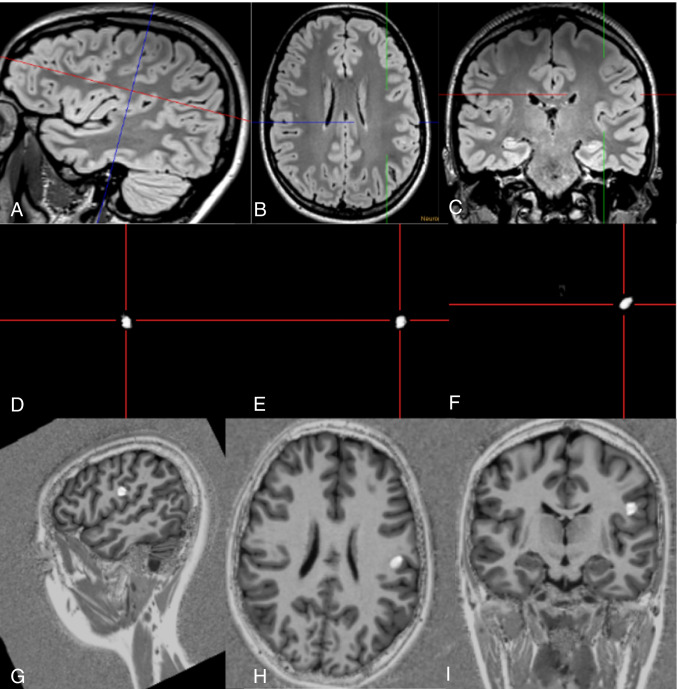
Subtle FCD of the left postcentral gyrus (#29). The lesion was detected on the 3D FLAIR sequence as it showed a subtle transmantle sign (A–C: crosshair). Junction images in sagittal (D), axial (E), and coronal (F) reformations showed a 0.33-ml large abnormality at the gray white matter junction. The co-registered MP2RAGE-ANN images confirmed the lesion (G–I). The patient underwent SEEG and subsequent surgery, confirming an FCD IIB
